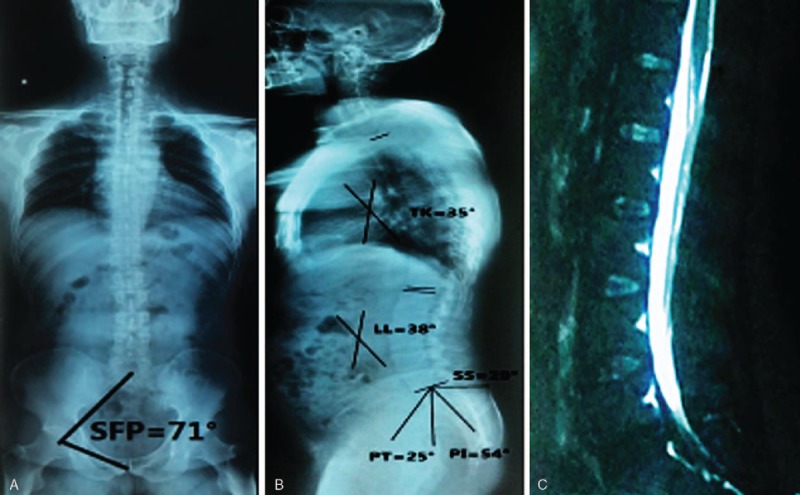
A male, 48 years old. SFP = 71°, PI = 54°, PT = 25°, SS = 29°, LL = 38°, TK = 35°. (A) A positive full spine X-ray; (B) lateral full spine X-ray; (C) lateral lumbar MRI showed no disc herniation. LL = lumbar lordosis, PI = pelvic incidence, PT = pelvic tilt, SFP = sacrum-femoral-pubic symphysis, SS = sacral slope, TK = thoracic kyphosis.
